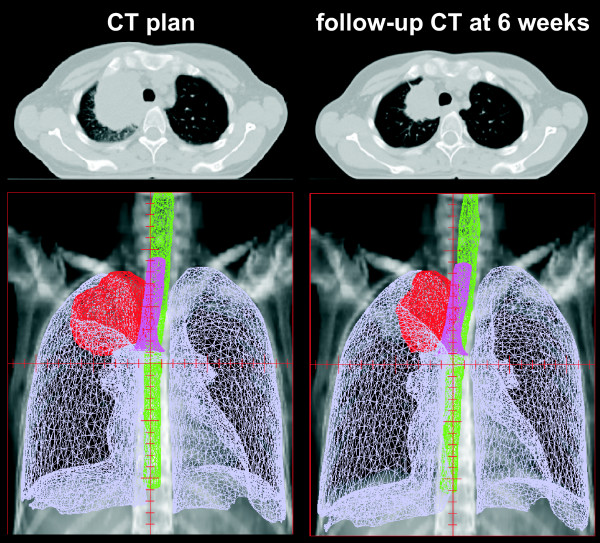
Planning CT image and follow-up CT image acquired at week 6 of combined radiochemotherapy for patient #6: corresponding three-dimensional meshes of the GTV, lungs, spinal cord and trachea are displayed in the second row.
